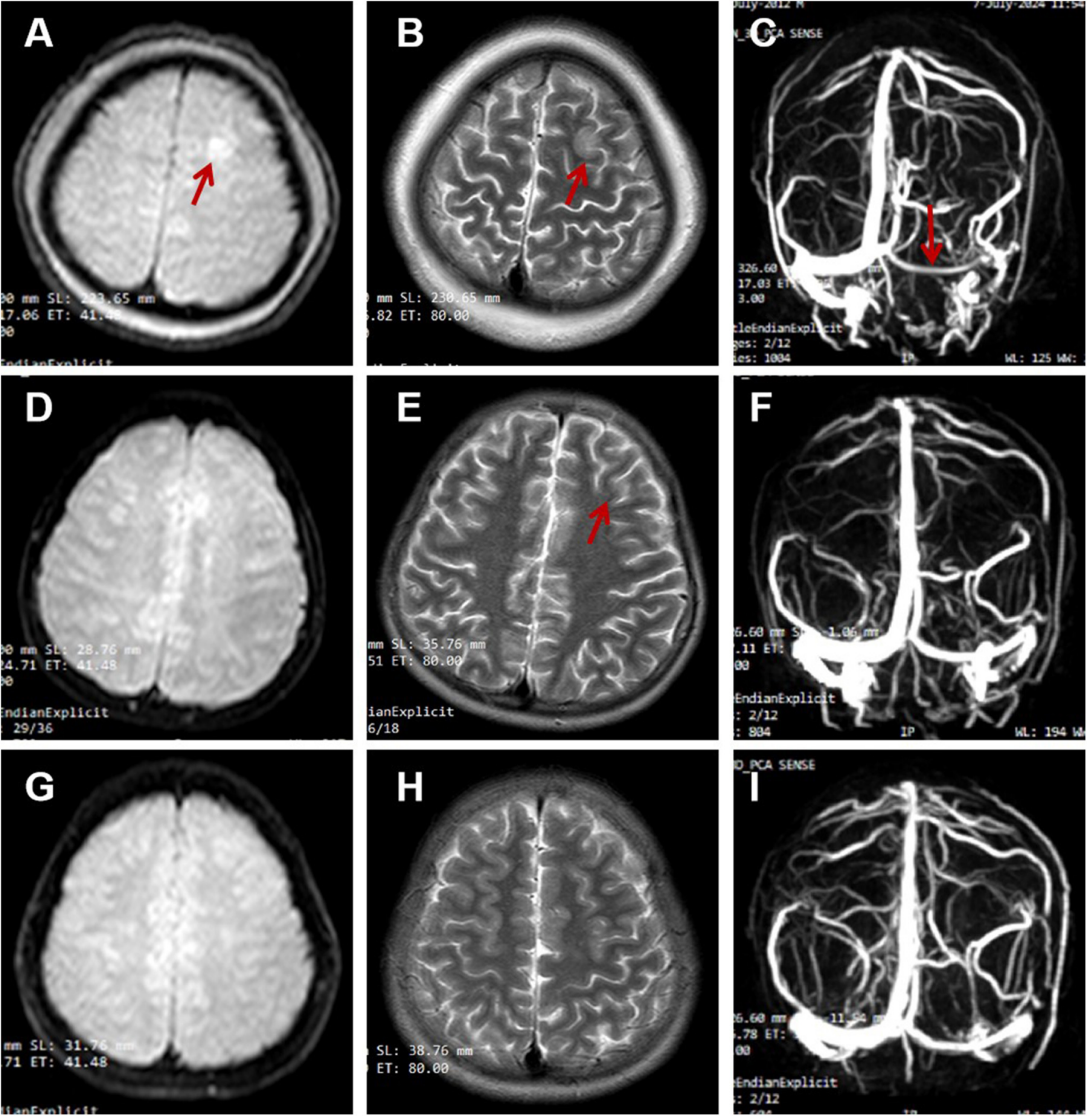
(A–C) on admission; (D–F) the second reexamination 16 days later; (G–I) the third reexamination 5 months later. (A) (DWI), (B) (T2-FLAIR) showed hyperintensity in the cortex and subcortex of the left frontal lobe; (C) (MRV) showed the reduced blood flow signal of the left transverse sinus. (D,E) showed the significantly shrunk of the infarct focus; (G,H) showed no infarct focus; (F,I) showed recanalization of transverse sinus.
